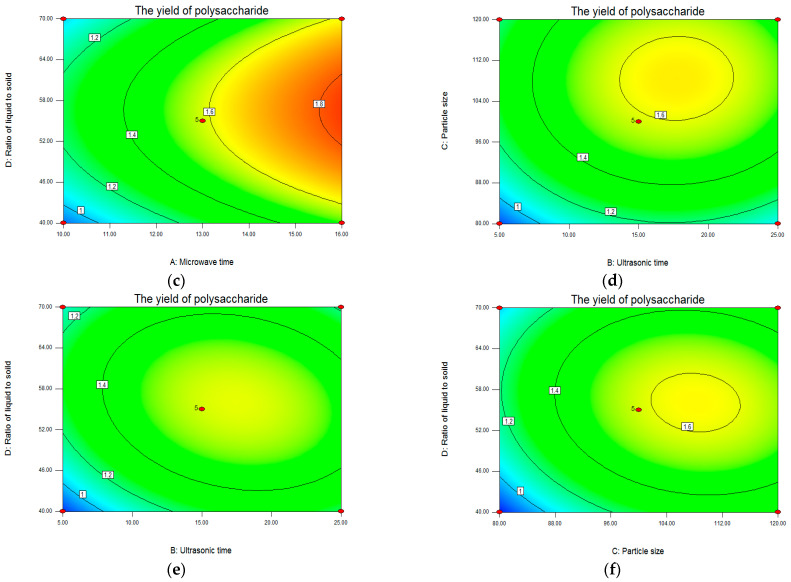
Contour plots of ultrasound–microwave combined extraction. (a) microwave time versus ultrasonic time; (b) microwave time versus particle size; (c) microwave time versus ratio of liquid to solid; (d) ultrasonic time versus particle size; (e) ultrasonic time versus ratio of liquid to solid; (f) particle size versus ratio of liquid to solid.
